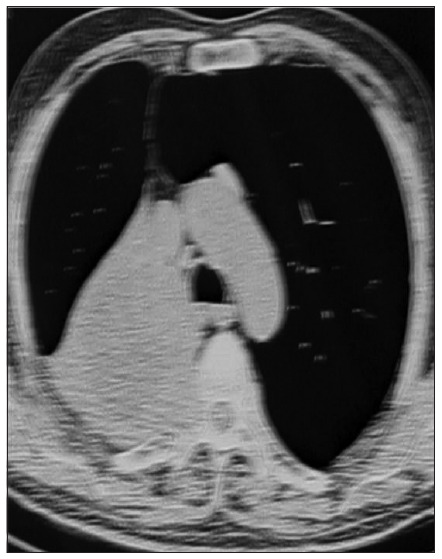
Chest computed tomography with contrast: a mass is seen in the right lung apex with destruction of the adjacent rib vertebra and extension to the spinal canal. Collapse of the right upper lobe is also seen, as a result of bronchial obstruction.
